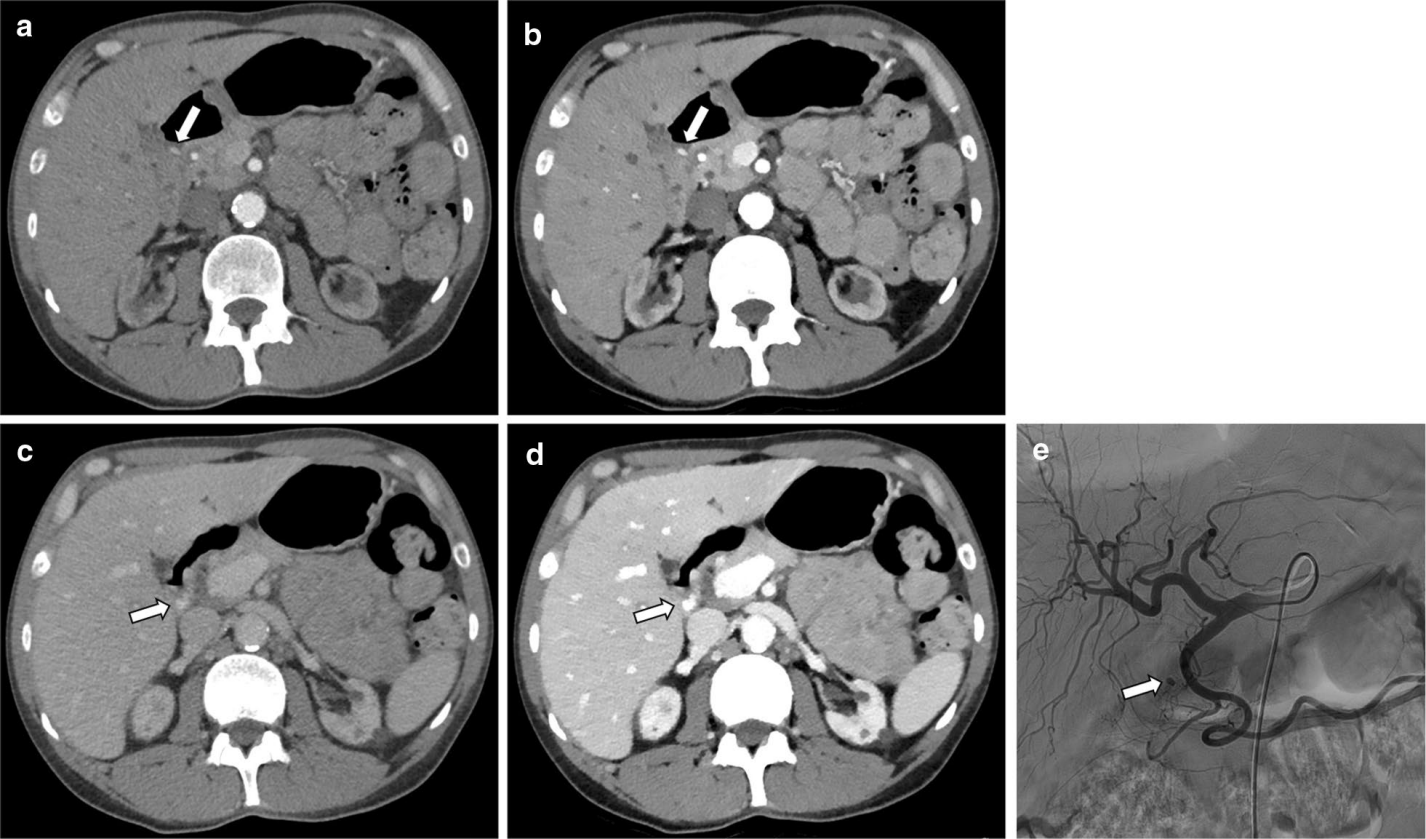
A 40-year-old man with upper gastrointestinal bleeding who underwent multiphasic CT for localization. Conventional images show contrast extravasation in the duodenal bulb (arrows) on the arterial (a) and venous (c) phases. The extravasation is more conspicuous using 50 keV mono-energetic images on both the arterial (b) and venous (d) phases. The focus of active bleeding is proven on digital subtraction angiography (e)
